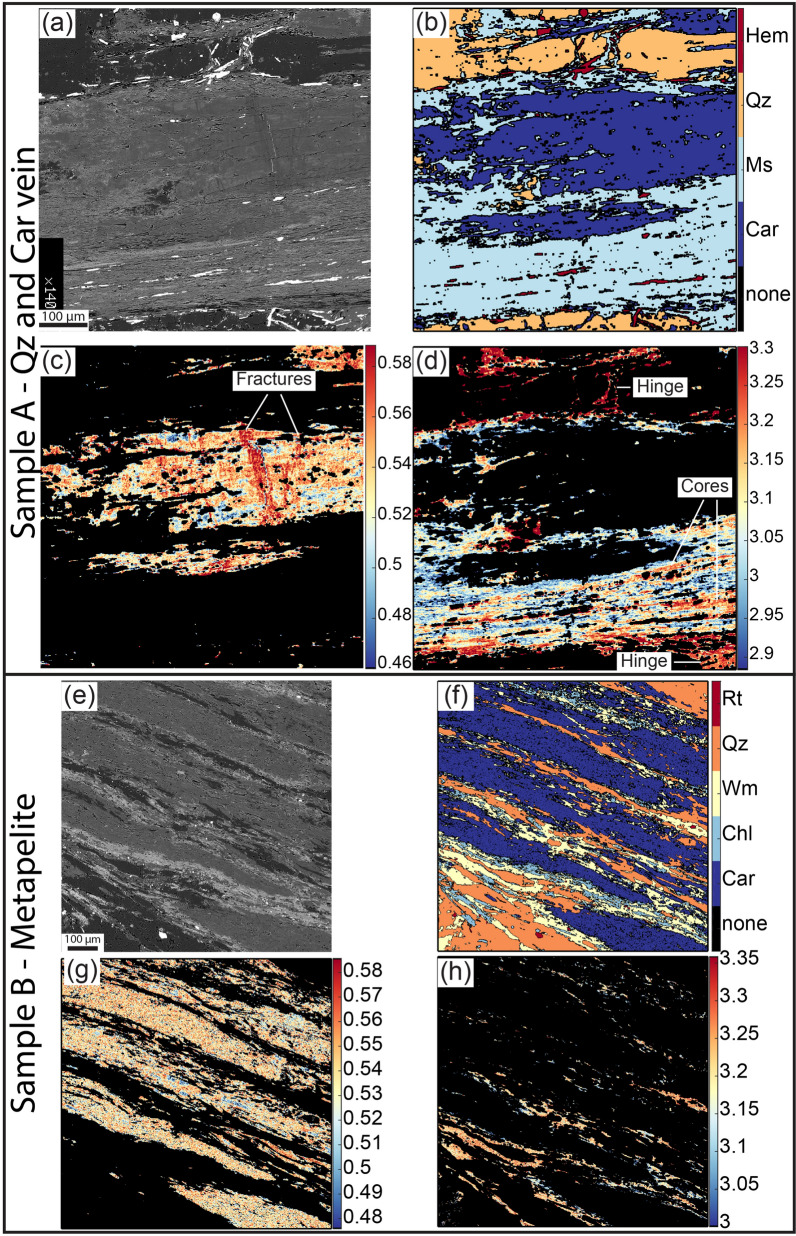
Chemical data from mineral phases infilling the dilational hydroshear veins and defining the mylonitic foliation. (a–d) Details of Fig. 3d,e. (a) BSE image showing fractures perpendicular to the carpholite fibre sealed by darker carpholite (compare with b and c). (b) X-ray compositional map, colour coded for the different mineral phases. (c) Standardised X-ray map of the X_Mg_ (X_Mg_ = Mg/(Fe^2+^ + Mg) in carpholite. Note the fractures oriented perpendicularly to the lengthening of the grain sealed by a second generation of carpholite with higher X_Mg_. (d) Muscovite Si apfu map highlights two muscovite generations: the first with higher values located in the core of the grains and in fold hinges and the second with lower values at grain boundaries. (e–h) Details of Fig. 3h,i. (e,f) BSE image and X-ray compositional map displaying a foliation marked by carpholite, muscovite and quartz, with chlorite growing at grain boundaries and marking retrogression. (g) Carpholite X_Mg_ map. (h) Muscovite Si apfu map. The lower values are located in proximity of chlorite grains.
